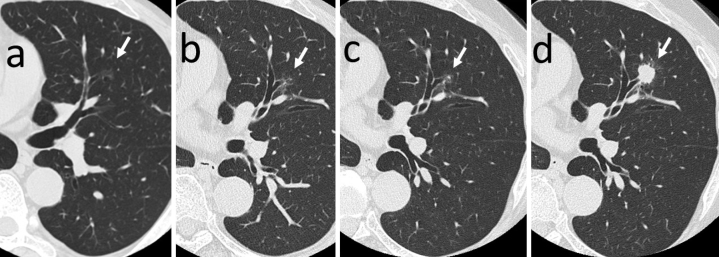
Transverse views of thin-section CT images in (a) 2007, (b) 2018, (c) 2019, and (d) 2020. The white arrows represent abnormal nodular shadows.
